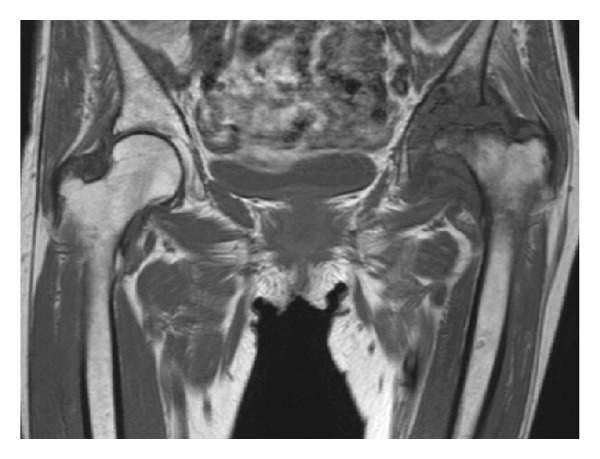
MRI T1WI at the time of admission to our hospital. Femoral head was completely collapsed and low band was observed in the upper edge of the femoral head.
